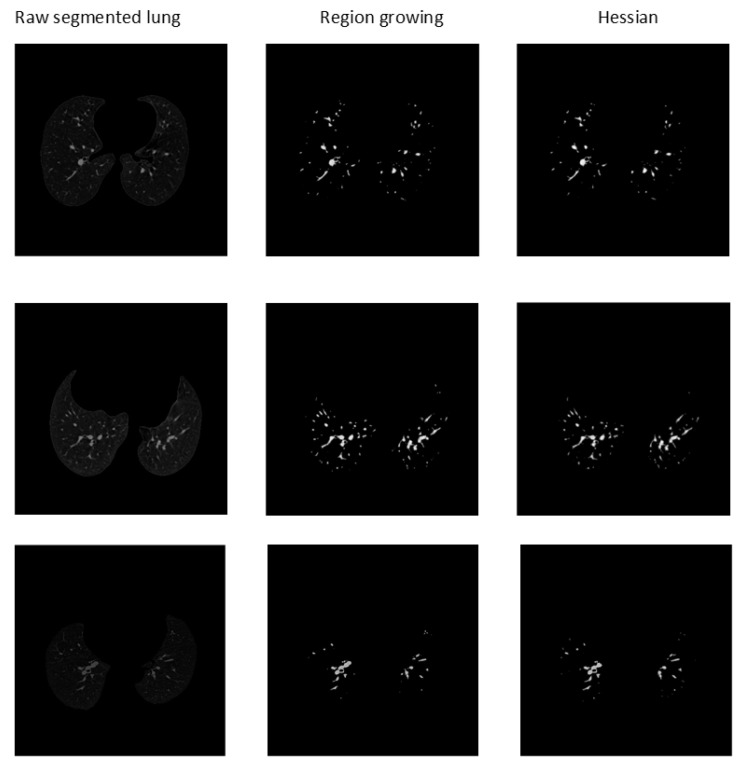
Segmented arteries from CT scans using region-growing and Hessian methods.
